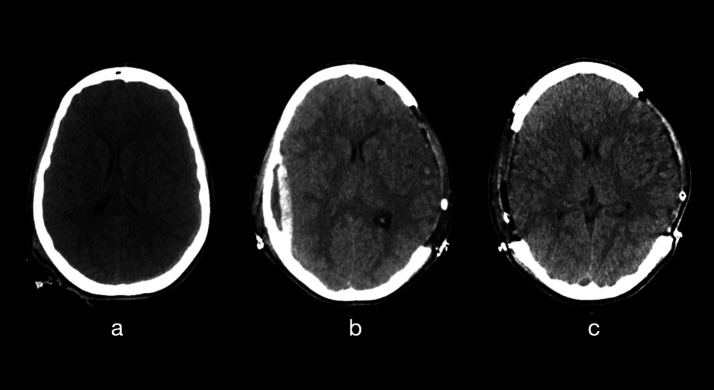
Non-contrast head computed tomography (CT) images of the patient. (A) Head CT scan at admission showing a left frontotemporoparietal acute subdural hematoma. (B) Postoperative day 1 CT demonstrating a newly formed contralateral right epidural hematoma. (C) CT after bilateral frontotemporoparietal decompressive craniectomy showing decompression and extensive bilateral calvarial defects.
